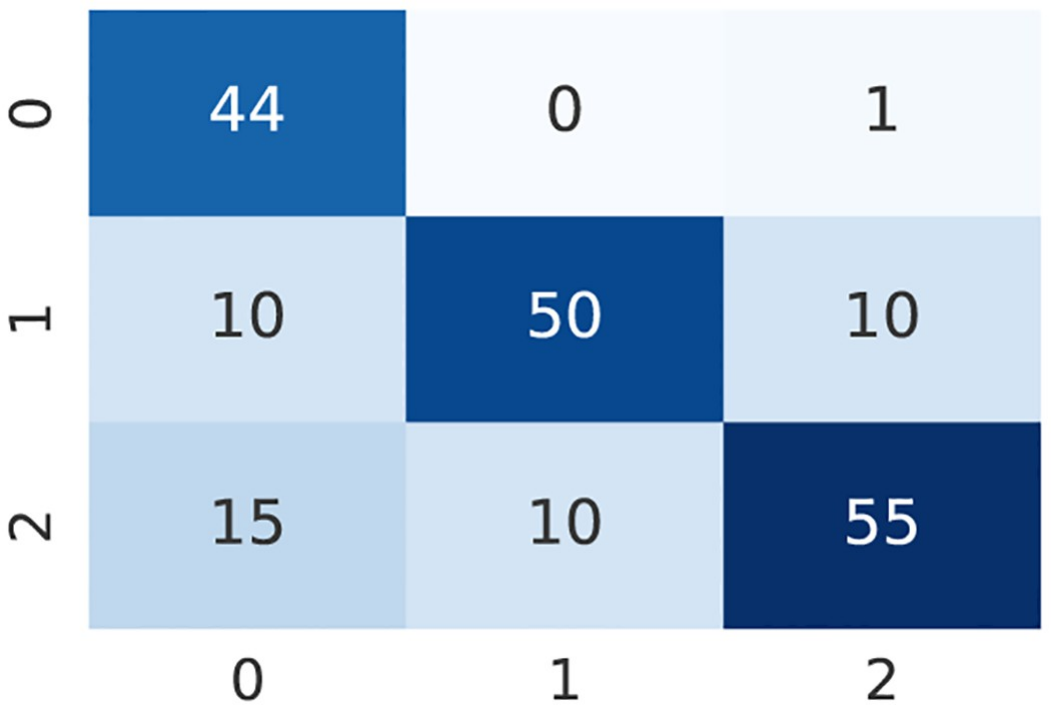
An example of confusion matrix. 0 denotes patients with normal DE-MRI, 1 patients with Myocardial Infarction, and 2 patients with Myocarditis.
